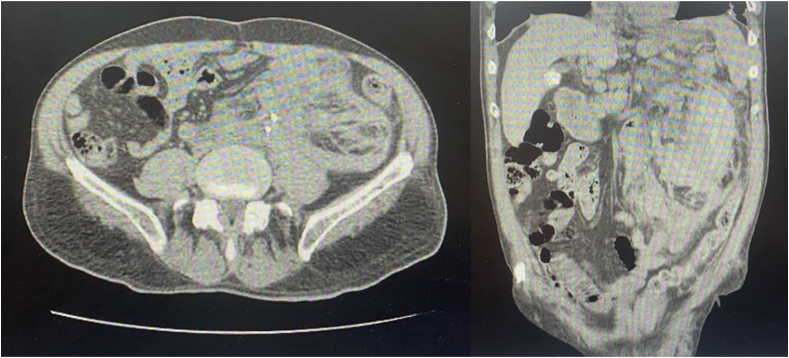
Transverse and coronal sections of the CT scan show a massive hematoma of the left kidney.
